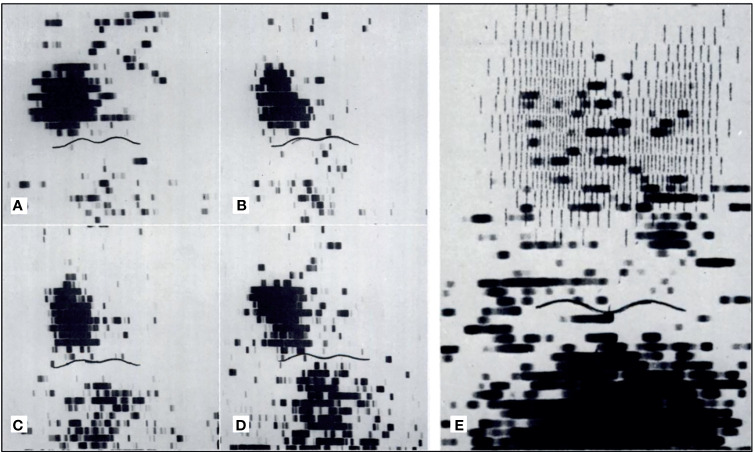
Early parathyroid scintigraphy using ^75^Se depicting a 2.3g parathyroid adenoma on the right. (A, B) 10 and 45 minutes after intravenous administration of radiotracer with tumor tracer localization and low tracer activity in the sternal region. (C, D) 2 h and 24 h after radiotracer administration with tumor tracer localization and increasing radiotracer activity in the sternal region. (E) Superimposition of thyroid scan onto parathyroid scintigraphy with 2.7 g parathyroid adenoma inferior to the left lobe of the thyroid. Reproduced with permission from: *Colella AC, Pigorini F. Experience with parathyroid scintigraphy. Am J Roentgenol Radium Ther Nucl Med. 1970 Aug;109(4):714-23. doi: 10.2214/ajr.109.4.714. PMID: 5451873*.
